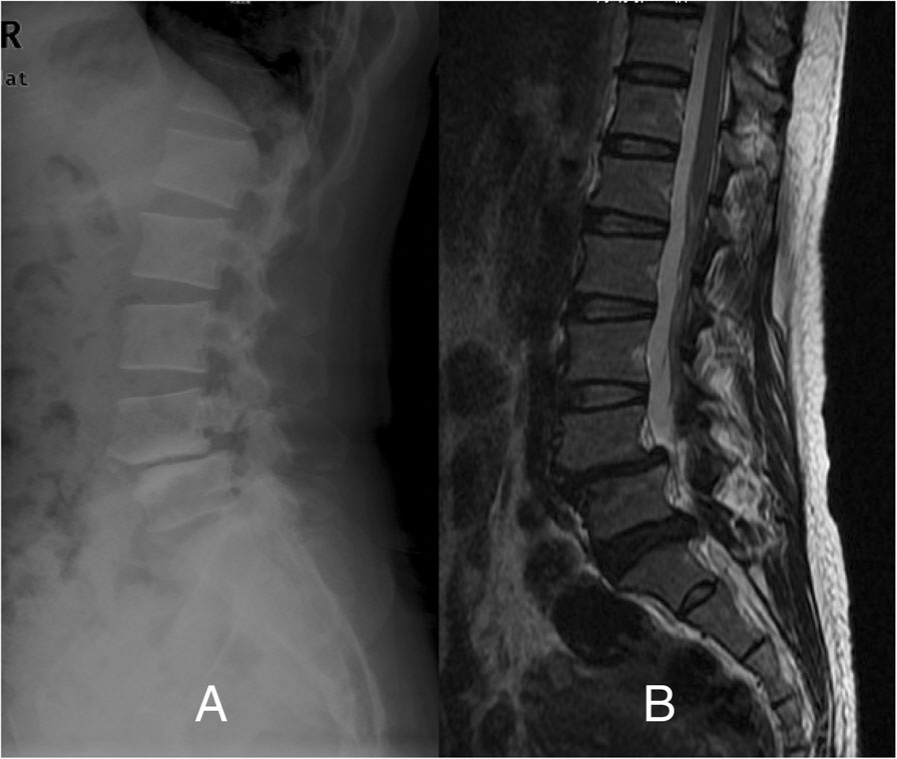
Preoperative lateral X-ray (a) and sagittal MRI (b) of lumbar spine demonstrated L4 spondylolisthesis and spondylolysis in both sides and disc protrusion of L5/S1 compressing left L5 and S1 root
